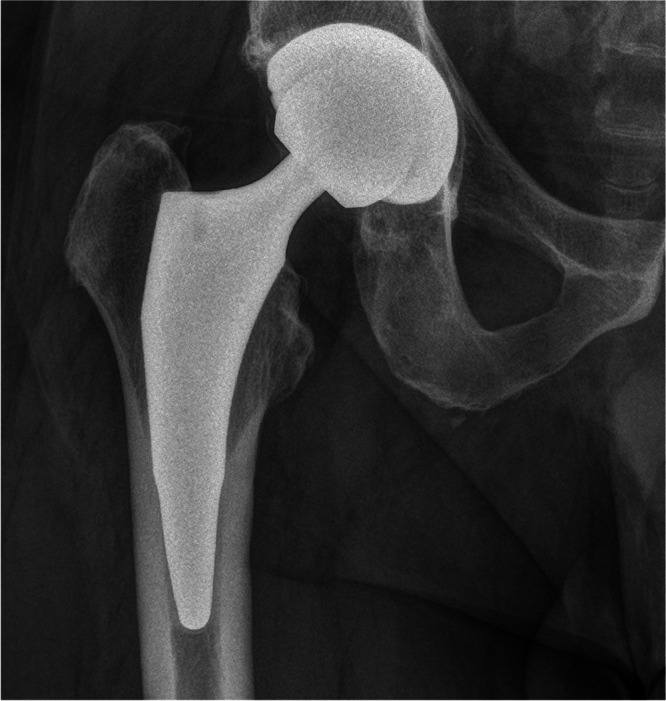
Two‐and‐a‐half‐year radiographic follow‐up showing stem radiolucent lines (RLLs) confined to Gruen Zone 4.
